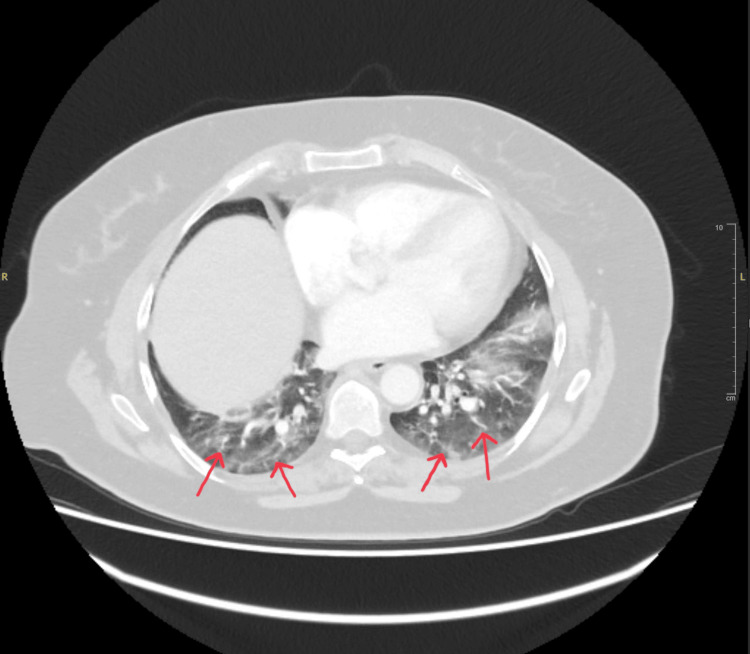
Cross-sectional CT of the chest and abdomen with contrast The red arrows indicate a significant dependent atelectatic change in both lungs with ground-glass attenuation in the lingula and both lower lobes.
